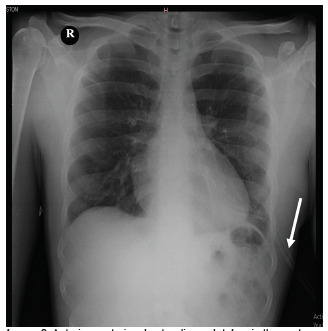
Anterior-posterior chest radiograph taken in the post-operative period for confirmation of the lung expansion. The chest tube was still in place (arrow).
